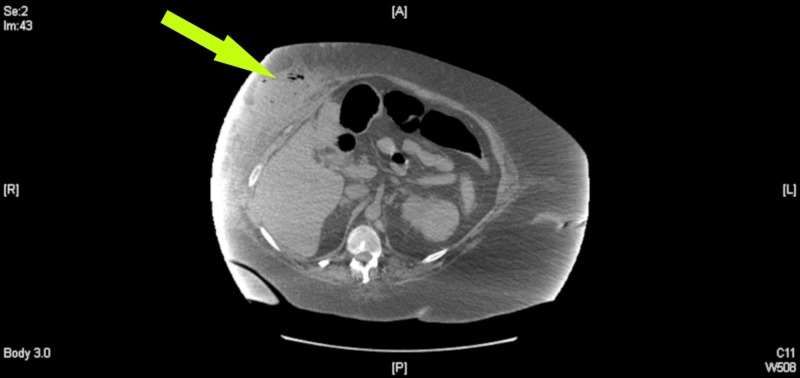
Axial view of noncontrast computed tomography of the abdomen. Arrow demonstrates subcutaneous right upper quadrant fluid collection with associated air-fluid levels and local fat stranding. Superiormost image of the abscess site.
